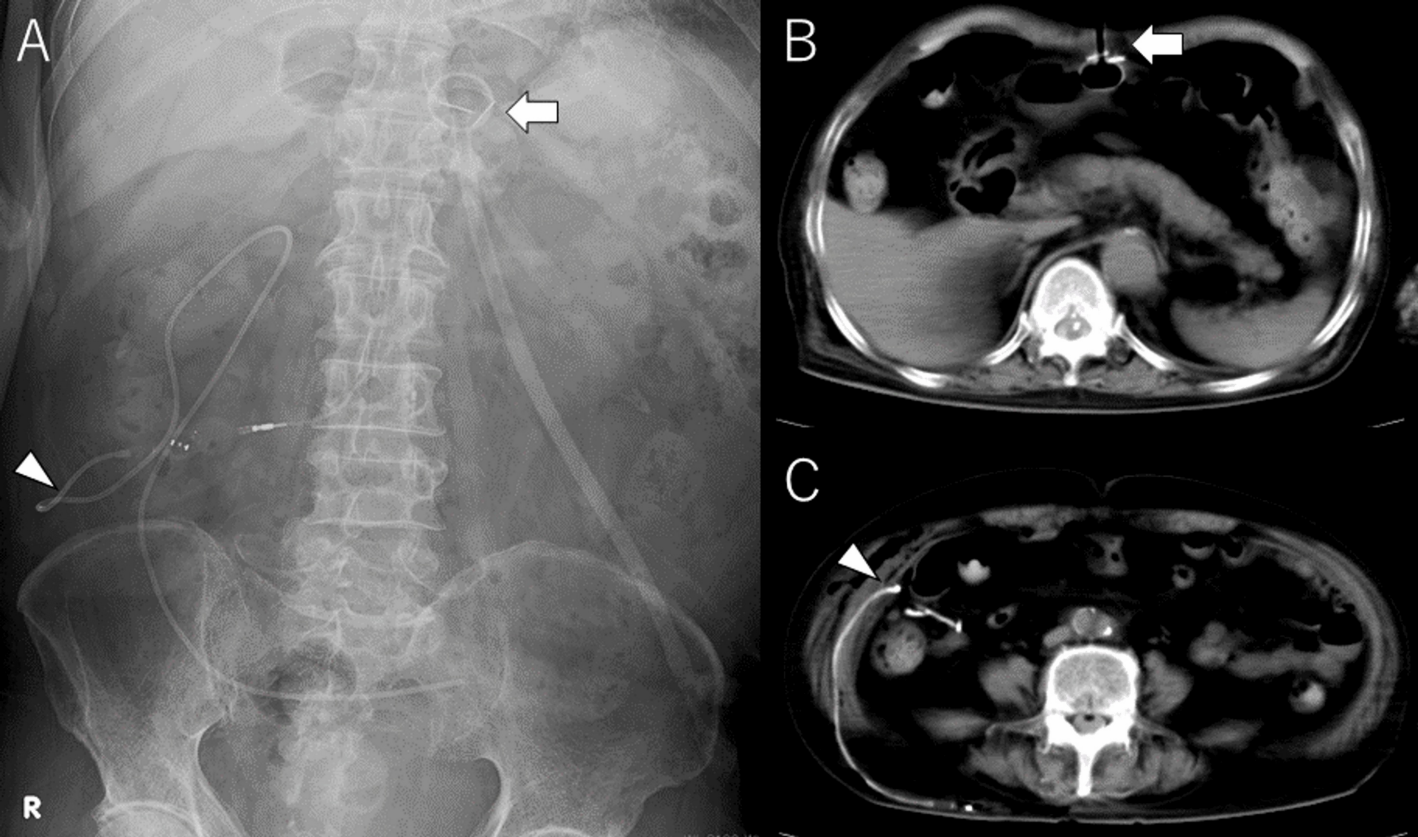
Images after PEG and LP shunt (A) Abdominal X-ray showing the position of the PEG tube (arrow) and the LP shunt insertion site (arrowhead). (B, C) Abdominal computed tomography after PEG and LP shunt placement showing the PEG tube in the upper abdomen (arrow) and the distal catheter passing between the internal oblique and transversus abdominis muscles, inserted into the right lower abdomen (arrowhead). PEG, percutaneous endoscopic gastrostomy; LP, lumboperitoneal
